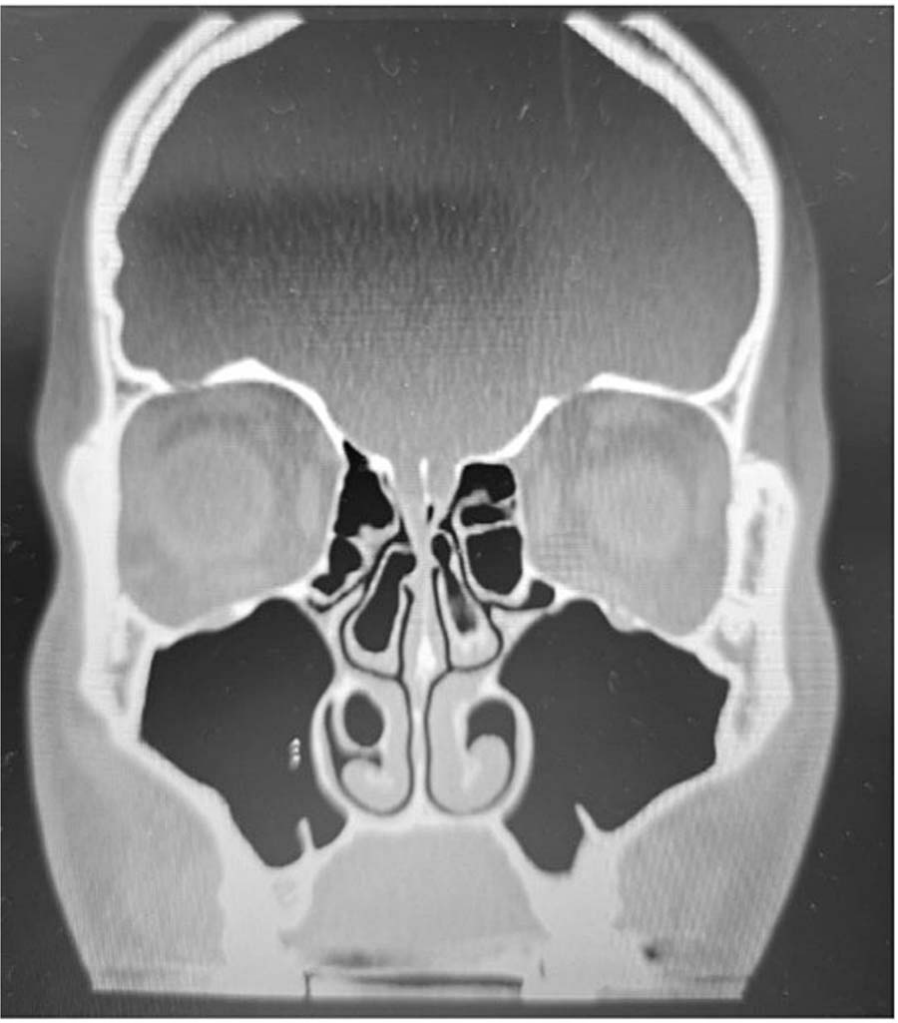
Paranasal sinus CT scan in the coronal plane showing bilateral concha bullosa in the superior turbinates and pneumatization of the right inferior turbinate (inferior concha bullosa).
